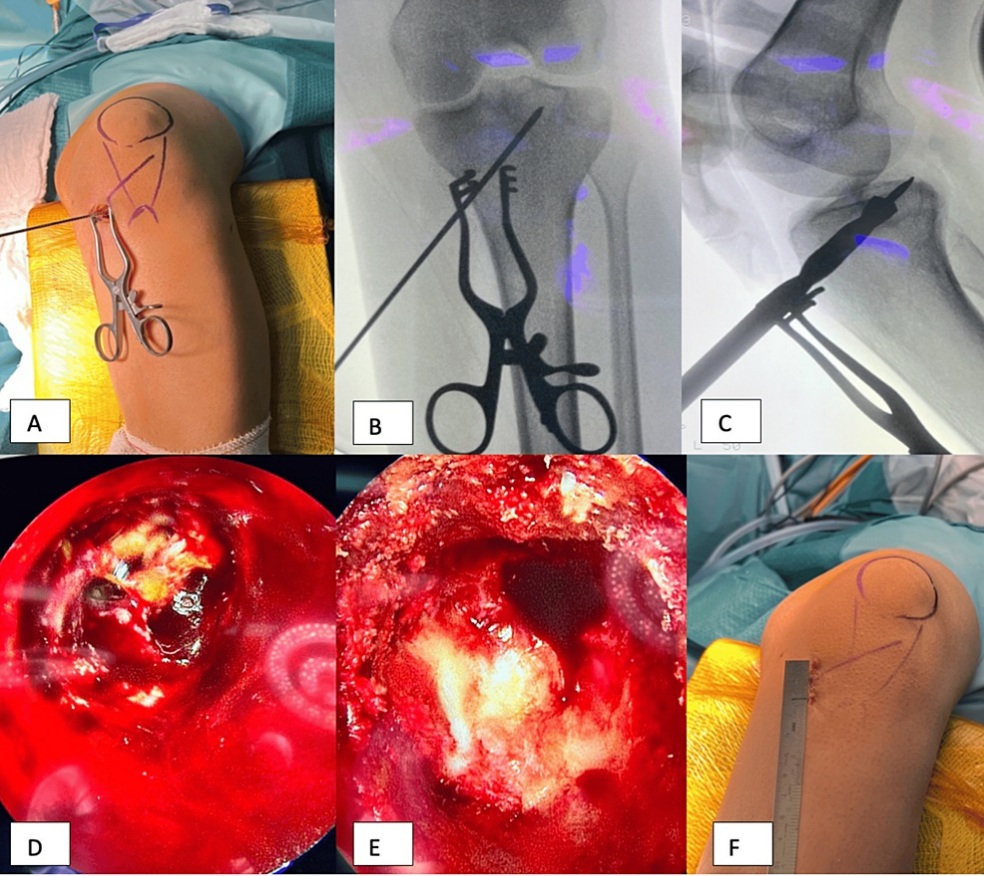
Surgical procedure. (A) Marking of the tibial tuberosity and skin incision. (B) K-wire was inserted and verified on AP and lateral views. (C) K-wire on the lateral radiographs with graduated ACL reamer. (D) Tumor as visualized by the endoscope, with brownish tumor tissue in the cavity. (E) Tumor cavity after curettage using curettes and irrigation; subchondral cartilage is seen on the cavity's roof. (F) Skin incision after closure. AP: Anteroposterior.
